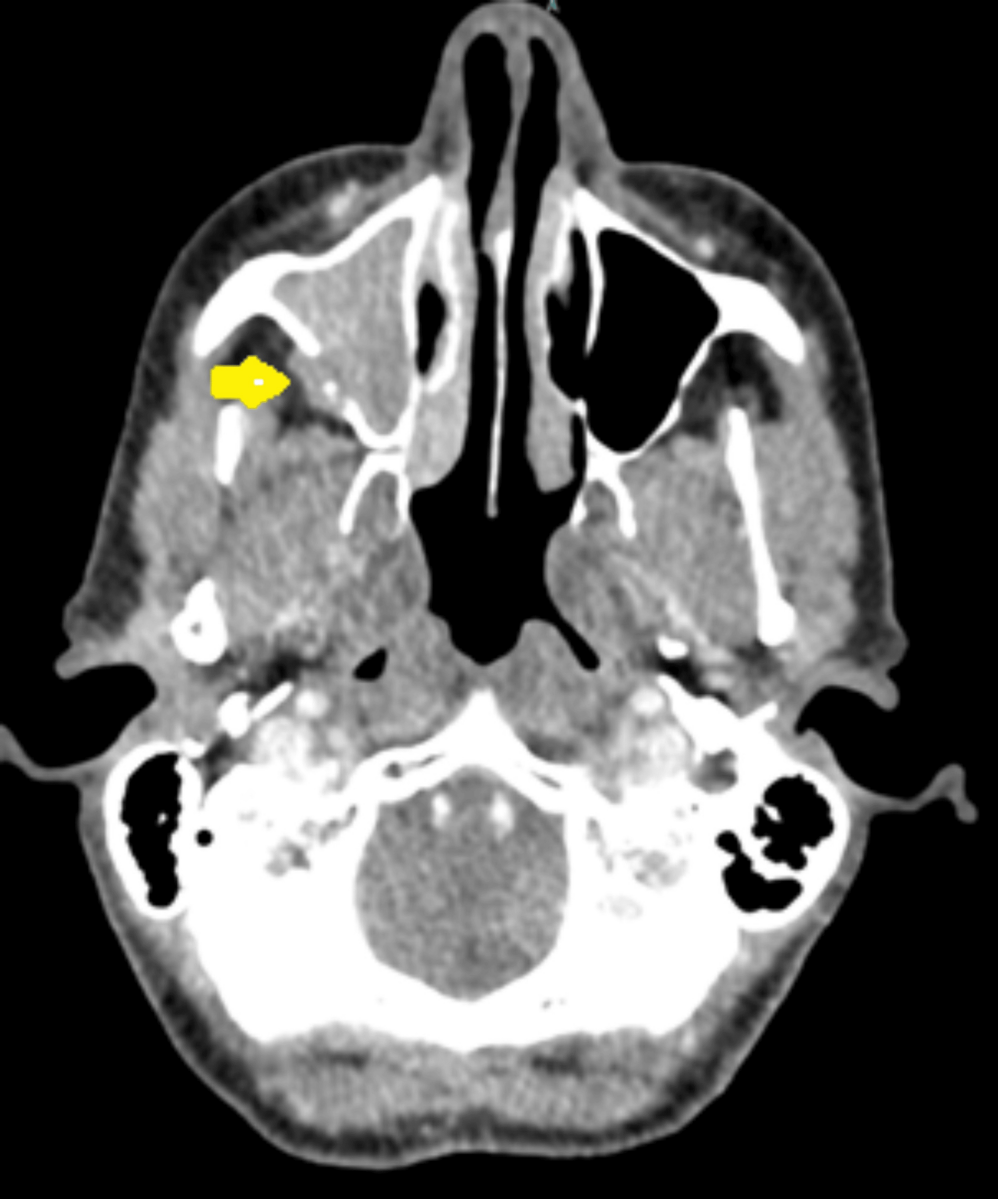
Contrast-enhanced CT in May 2022 (Axial view) Recurrent tumour filling the right maxillary antrum breaching the posterior wall and extending into the retro maxillary fat (yellow arrow).
